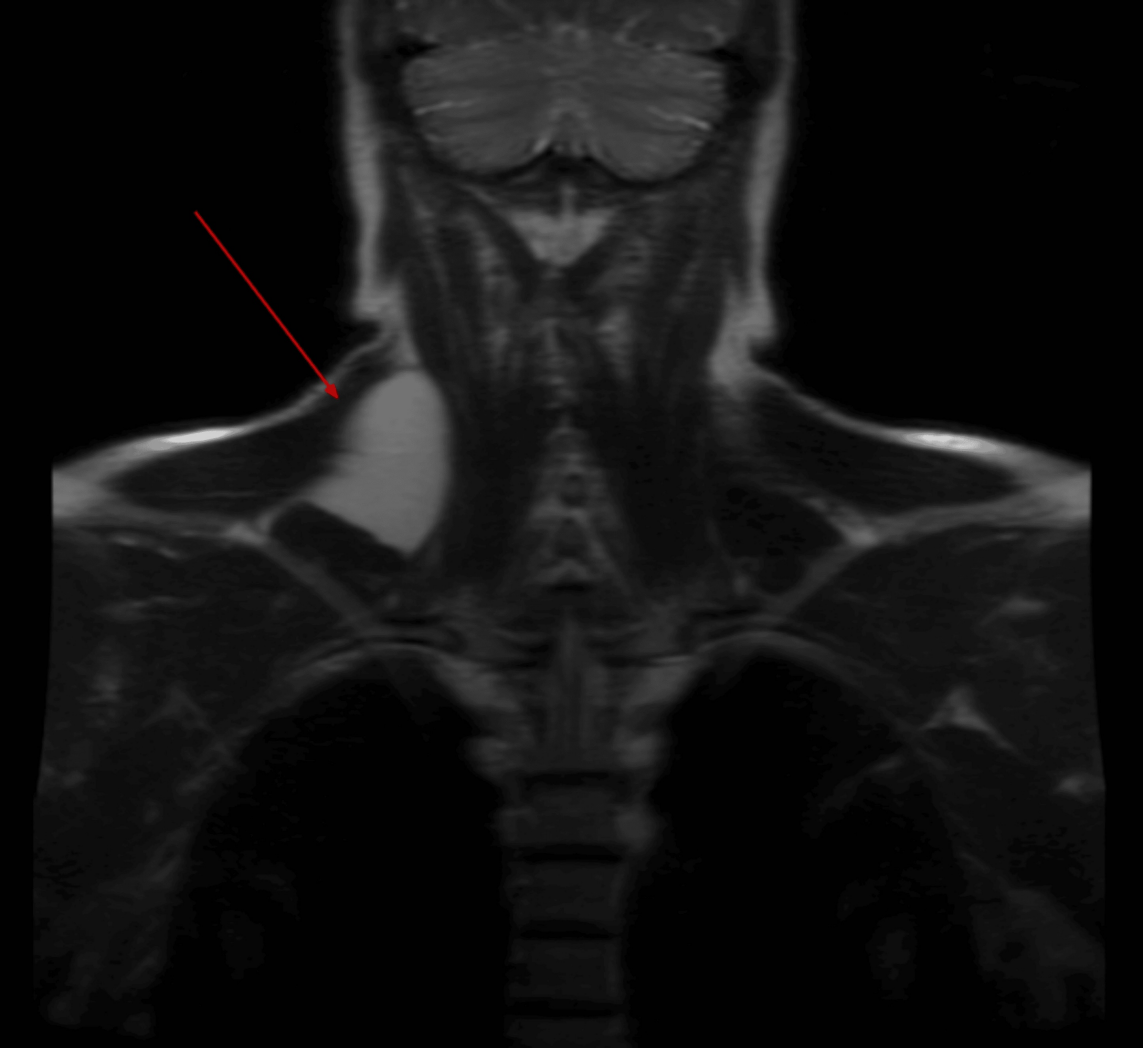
MRI cervical spine without contrast Deep right trapezius lipoma (red arrow) measuring 5.8 cm x 2.6 cm x 5.8 cm at the level of the cervicothoracic junction.
